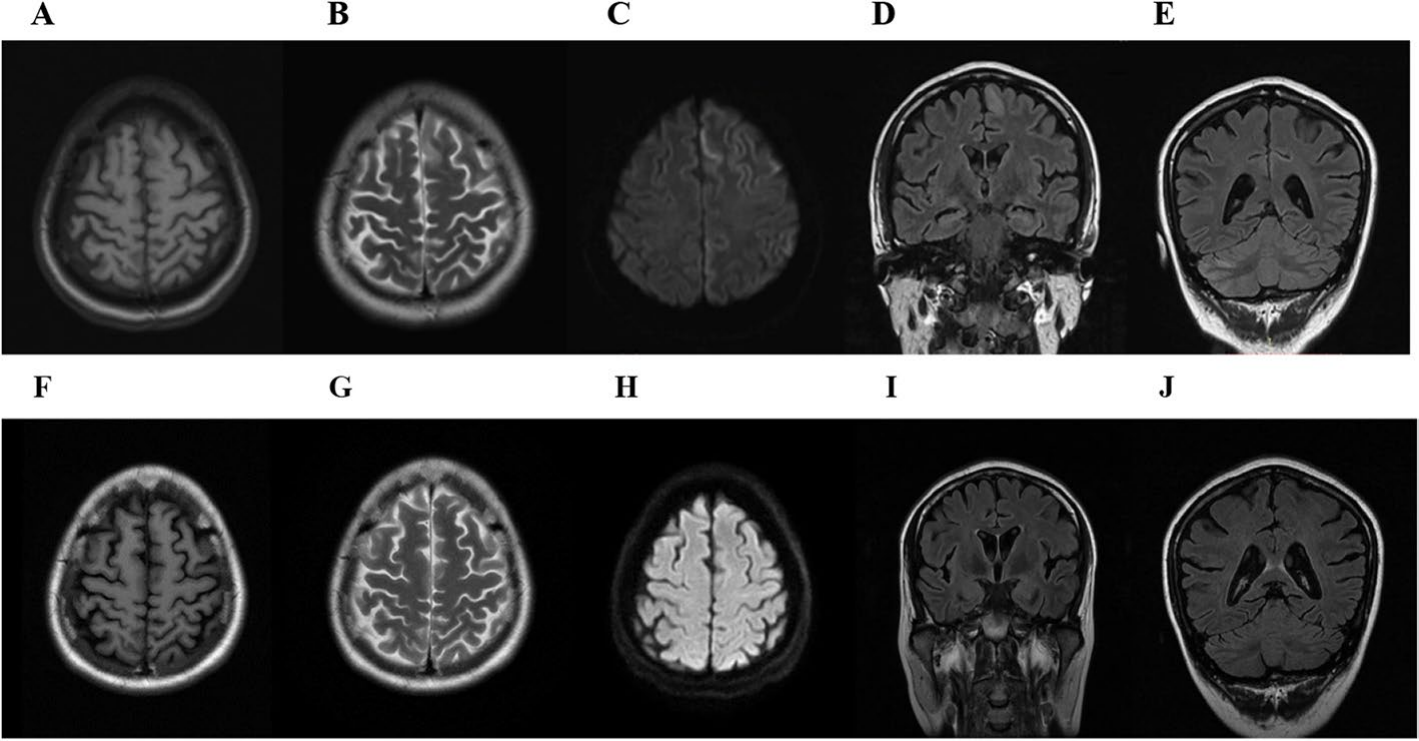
Brain MRI of the patient on 10–2020. A-D Abnormal signal in the left frontal lobe (white arrow). E Hyperintensity of the right cerebellum on FLAIR (yellow arrow). Brain MRI on 11–2020. F-J All the above abnormal signals disappeared
